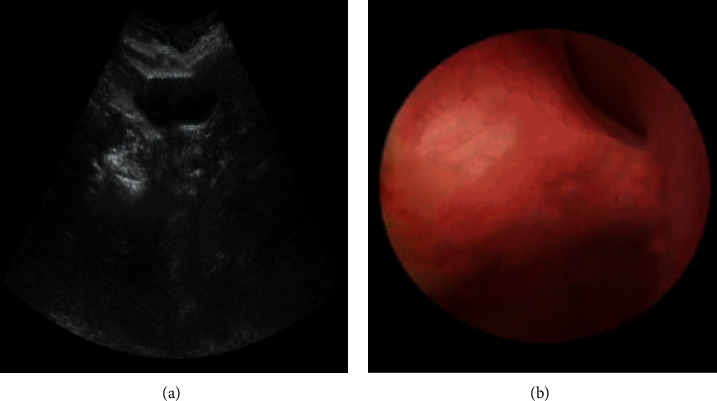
(a): Ultrasound image of submucous myoma of uterus; (b) hysteroscopy image of submucous myoma of uterus.
